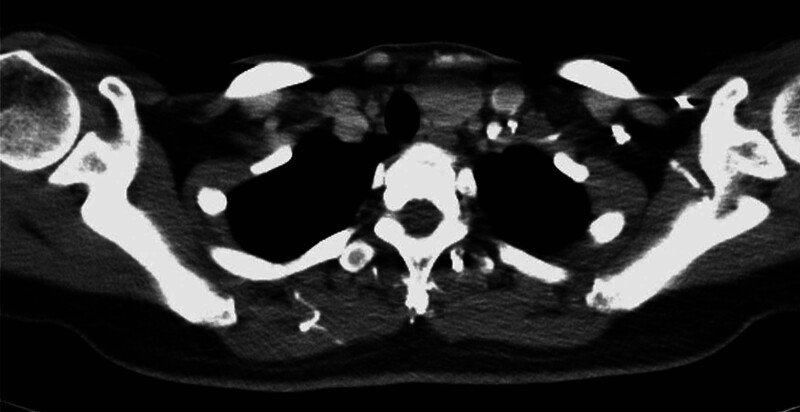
Computed tomography (CT) scan revealed a 3.8-cm mass situated within the left thyroid lobe without apparent signs of regional lymphadenopathy.
